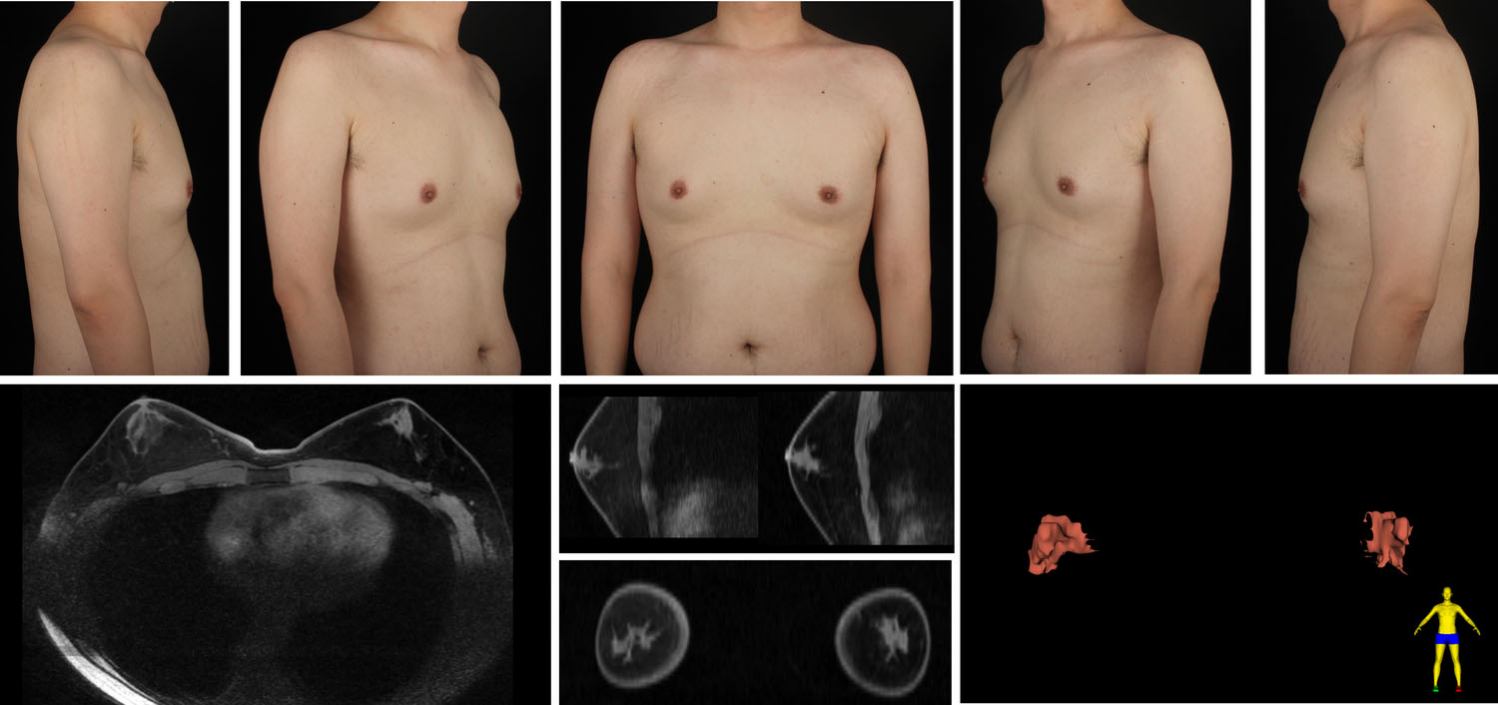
Type 2B gynecomastia. Preoperative chest appearance (upper) and breast MR images (lower) of a 33-year-old man with type 2B gynecomastia
